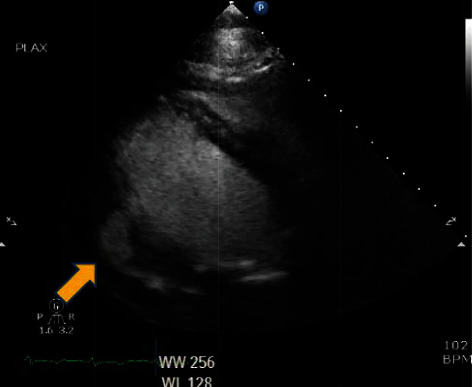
Parasternal long axis view of contrast leaking into the pericardial space on echocardiogram.
